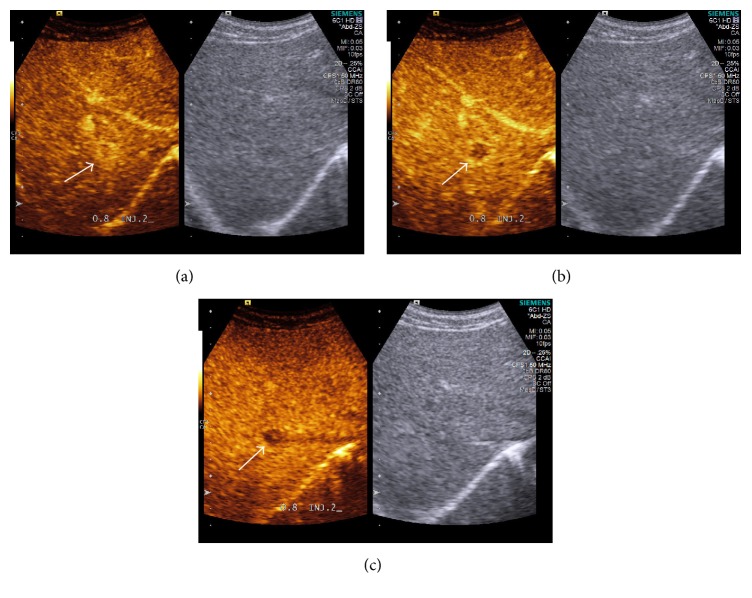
Small metastasis lesion from colon (15 mm) not clearly visible on B mode ultrasound showed rim-like hyperenhancement during arterial phase (a). Clear hypoenhancement during the portal venous (b) and late phase (c) on contrast-enhanced ultrasound. The arrow in the figure refers to rim-like hyperenhanced small metastasis lesion during arterial phase and showed clear hypoenhancement during the portal venous.
